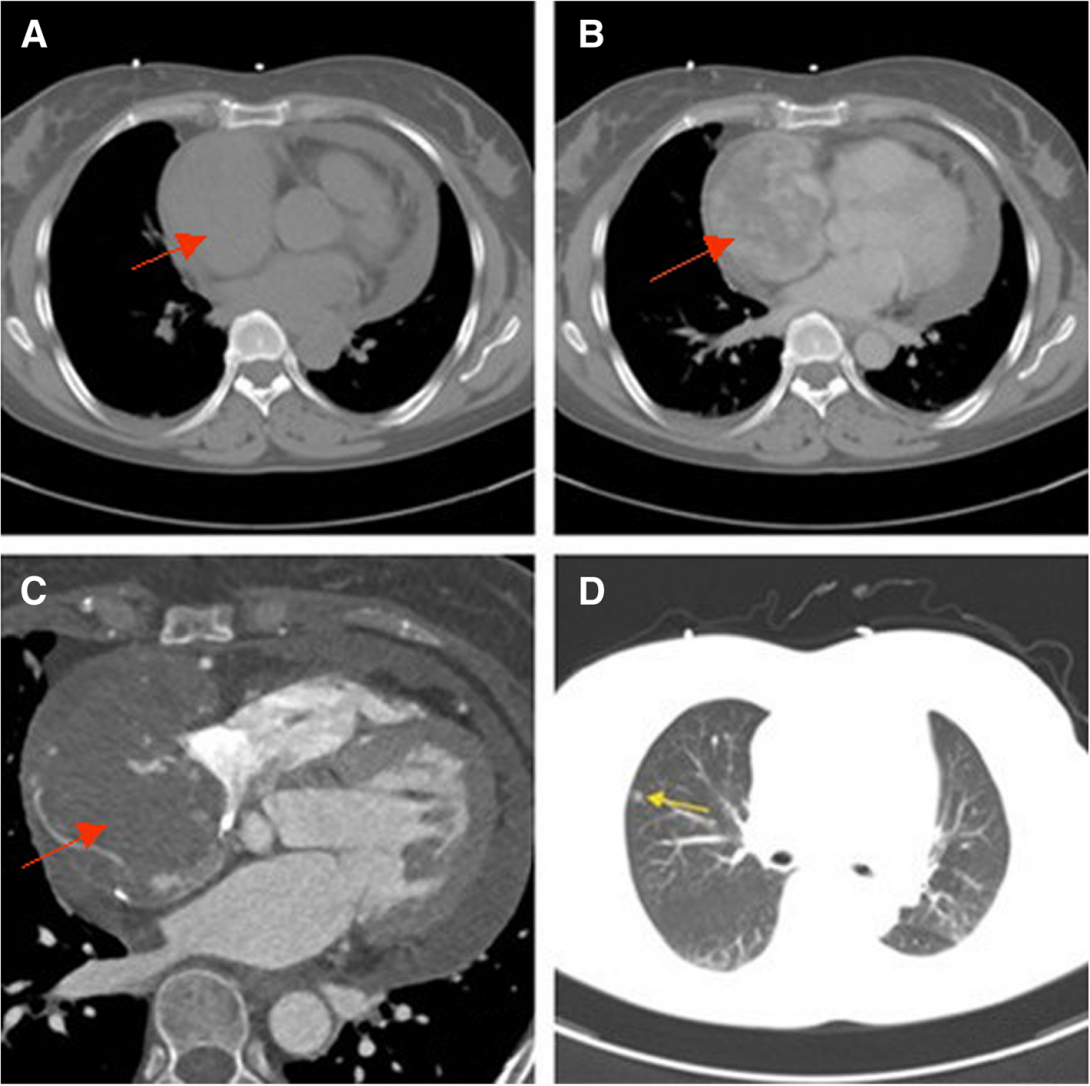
Female, 56 years old, right atrium angiosarcoma (red arrows). a: Plain CT scan shows an equal-density mass in the right atrium. The density is partly homogenous, and pericardial and pleural effusion are noted. b, c: Enhanced CT scan indicates inhomogeneous mass enhancement and an angiogram of tortuous small blood vessels. d: Metastatic nodules (yellow arrows) are seen in the right lung
